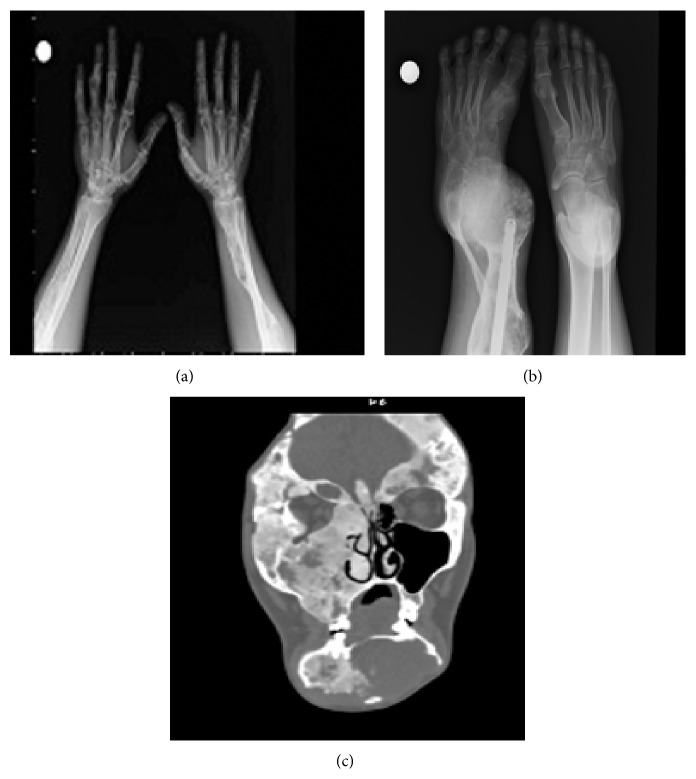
Radiologic images of patient 1. (a) X-ray of hands and forearms. (b) X-ray of feet. (c) CT images of craniofacial bones.
